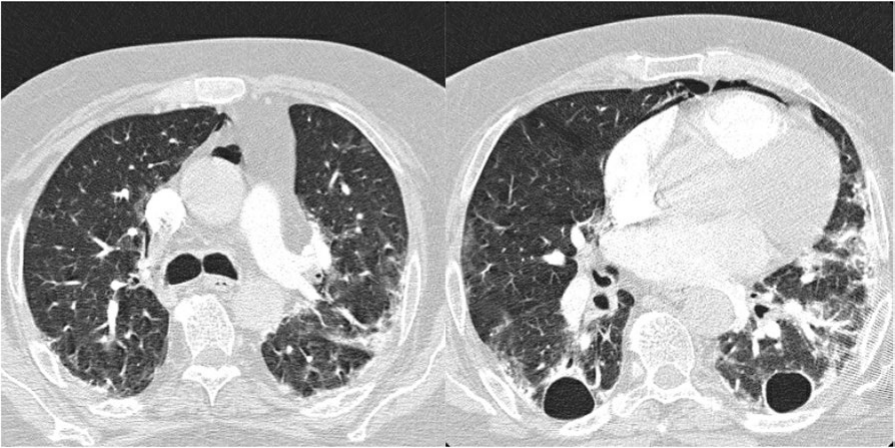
Axial sections of CT angiography of the pulmonary arteries, performed a month after the previous chest CT (Fig. 3), show progression of bilateral cavitary lung lesions and more evident traction bronchiectasis. It also shows development of bilateral peripheral patchy ground glass opacities and compressive atelectasis. Note is also made of pneumomediastinum
